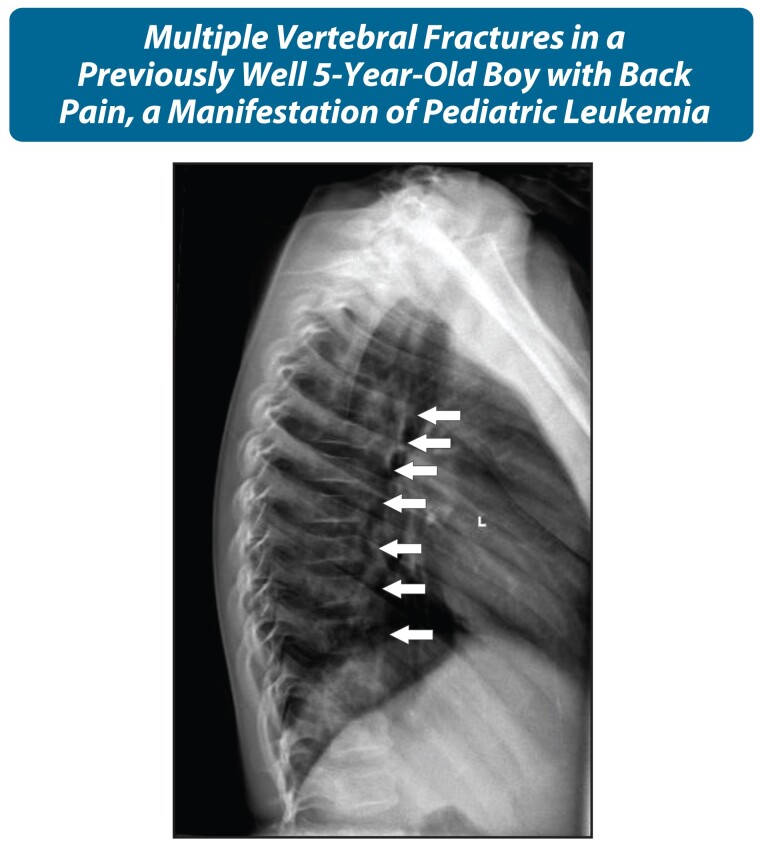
A lateral spine x-ray in a child with painful vertebral fractures due to leukemia, a known manifestation of hematological malignancy that is the diagnosis to rule out urgently. Interestingly, this patient also had a loss of function variant in the *LRP5* gene, which may have exacerbated his risk for low BMD and vertebral fractures. In addition, his CBC was not typical for leukemia at diagnosis (lack of hyperleukocytosis or suppressed marrow activity otherwise), raising the importance of painful vertebral fractures as a hallmark sign of leukemia. Longitudinal studies have shown that nearly 80% of children with vertebral fractures during leukemia therapy will undergo complete vertebral body reshaping without bisphosphonate therapy, with fracture severity (degree of vertebral height loss), fractures in the lumbar region, and residual growth potential negatively influencing the potential for reclamation of normal vertebral dimensions. Abbreviations: BMD, bone mineral density; CBC, complete blood count; *LRP5*, low-density lipoprotein receptor-related protein 5.
